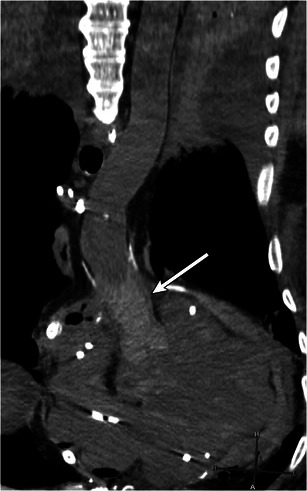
Arterial stasis thrombus. Non-contrast reconstructed CT image in a 67-year-old male on VA ECMO after a failed heart transplant demonstrates intraluminal high attenuation material in the proximal tubular ascending aorta compatible with thrombus
